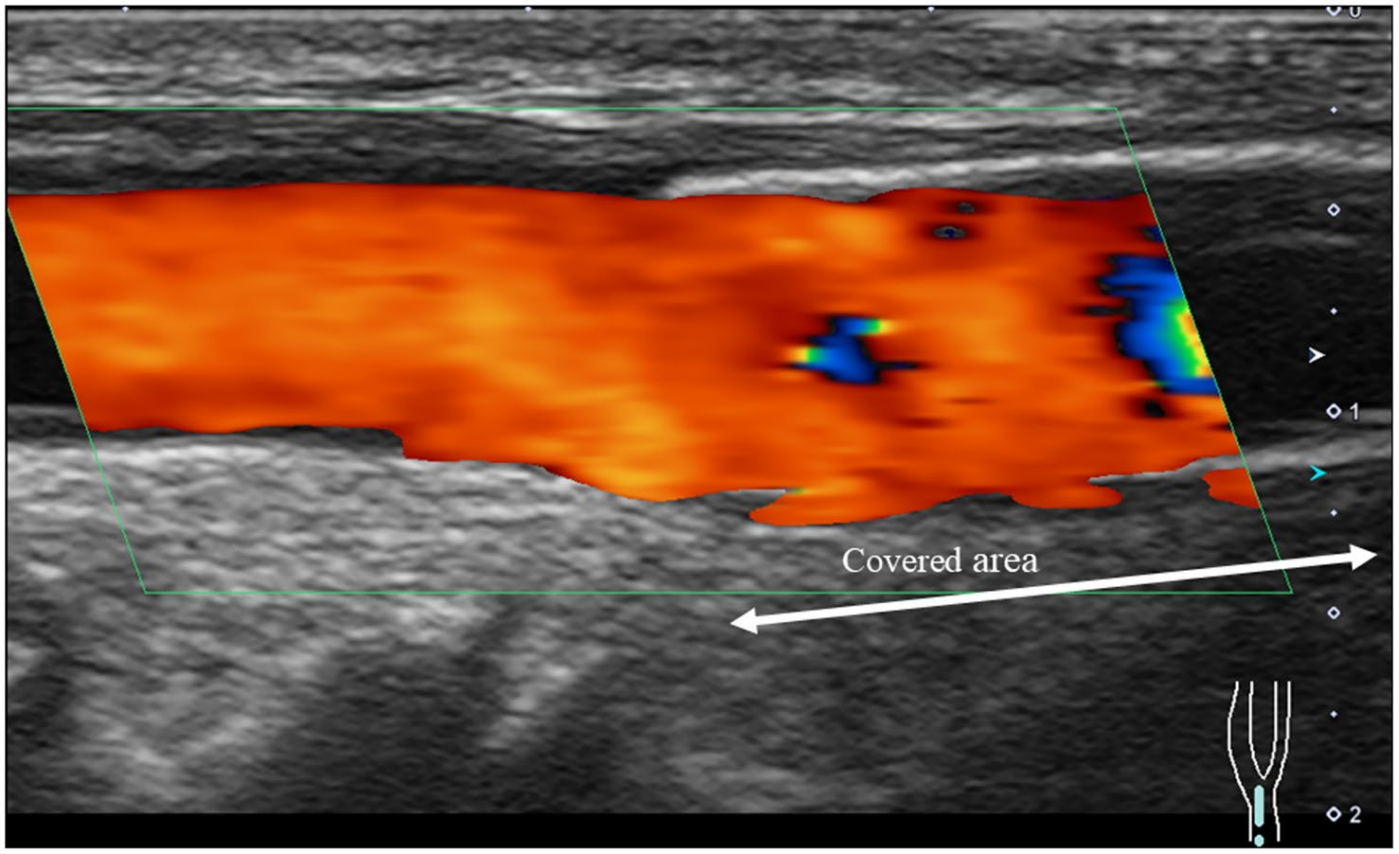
Condition of covered stent 4 years after surgery. Ultrasound image. The blood flow was maintained inside the stent.
